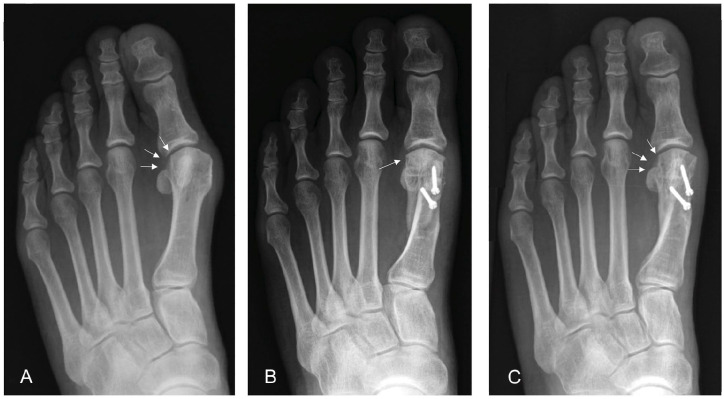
Dorsoplantar weightbearing foot radiographs showing the changes in the round
